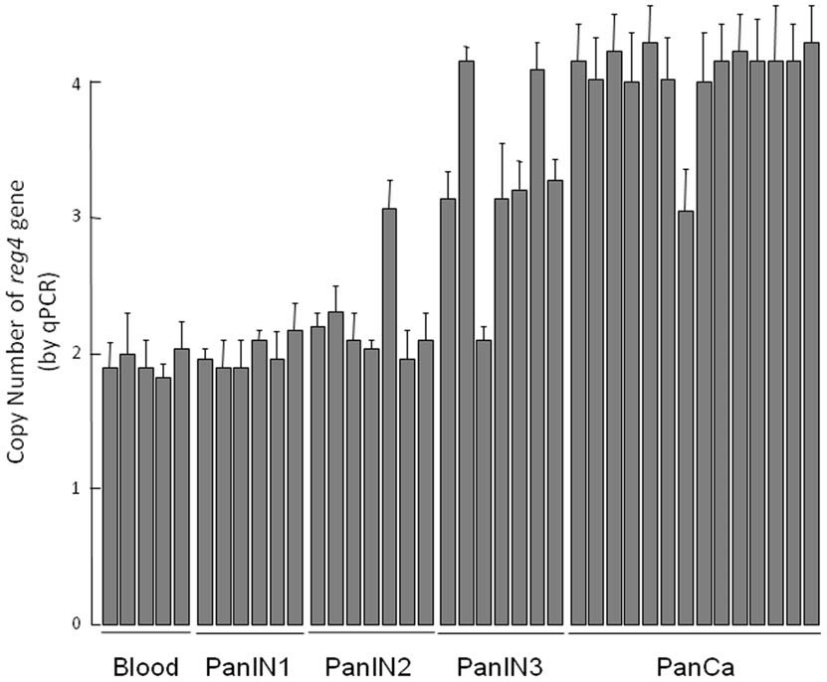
reg4* gene amplification in pancreatic cancer and precancerous lesions measured by quantitative PCR. Homogeneous populations of cells were carefully microdissected using a Laser Capture Microdissection System. PanINs were graded 1 to 3 as a function of the importance of architectural and cytological abnormalities. DNA from pancreatic cancer samples obtained by endoscopic ultrasound (EUS)-guided fine needle aspiration was used. DNA from blood leucocytes was used as control. *reg4* gene copy number was determined by qPCR performed in triplicate and results were analyzed using the RealQuant software.
